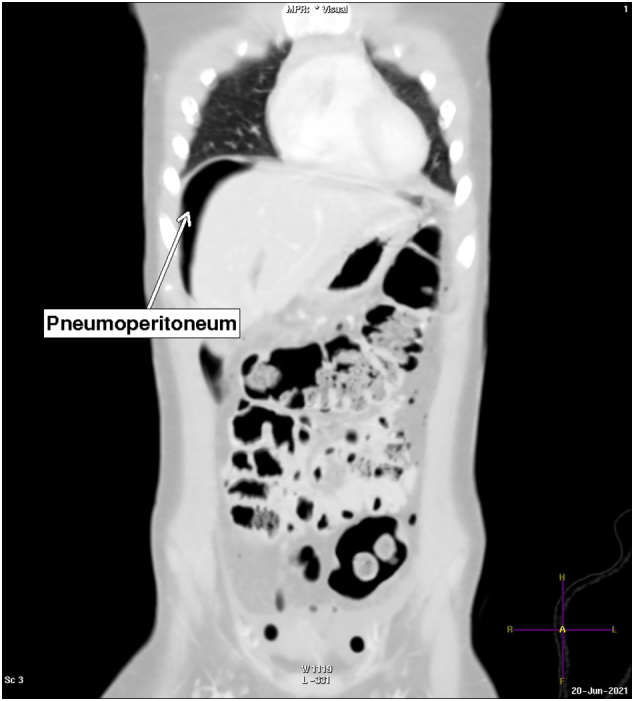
Coronal CT image in a lung window setting, free air around the liver margin and small specks of air foci on both sides of the abdomen.
